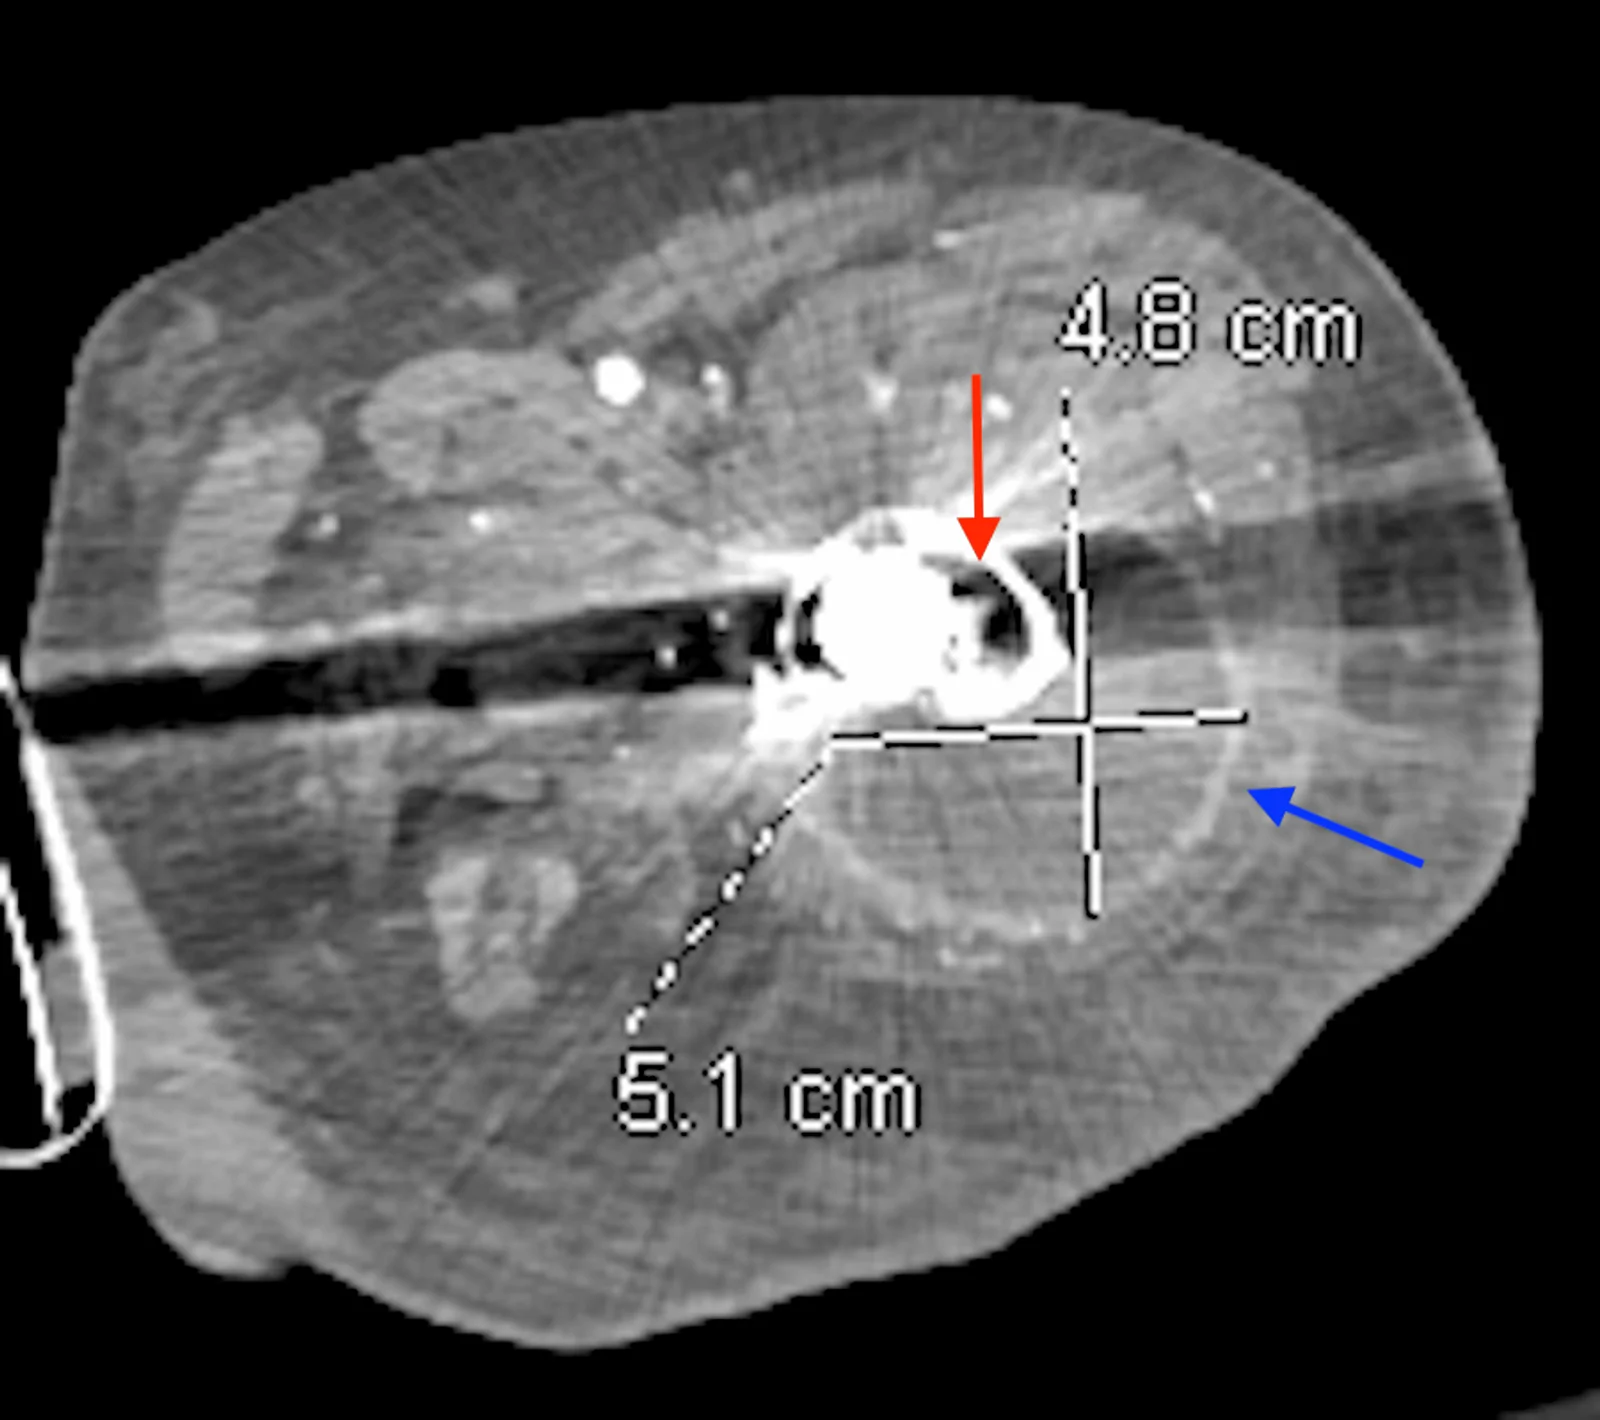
Axial CT angiography on soft tissue window. The image reveals left-sided periprosthetic lucencies (red arrow) and a fluid collection with an enhancing rim (blue arrow) directly posterolateral to the left proximal femoral diaphysis.
